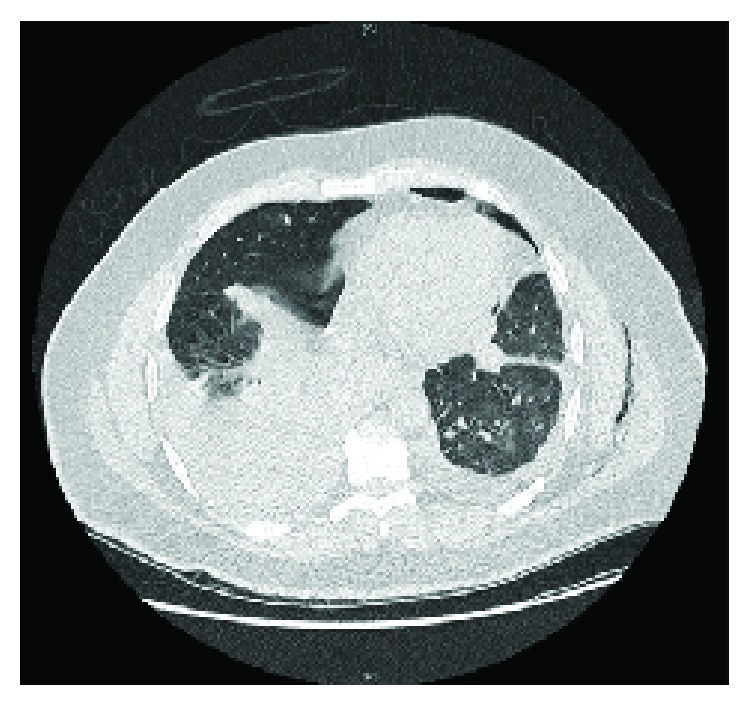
CT chest of the patient (axial view) showing the position of the chest tube and location of hemothorax.
